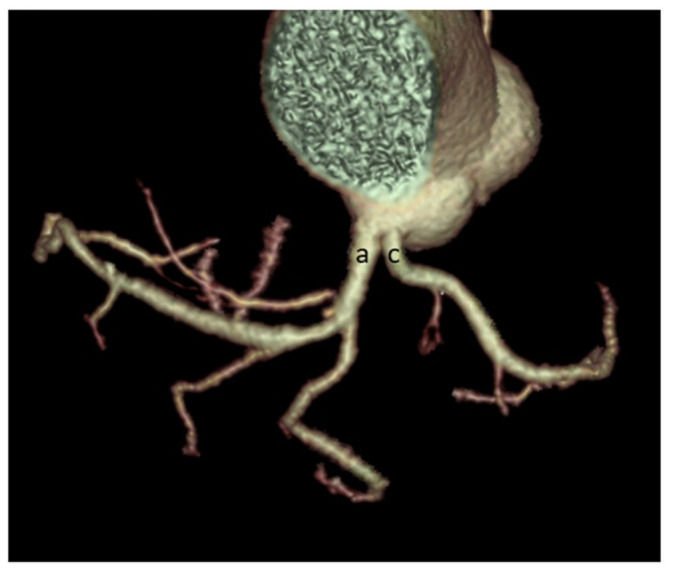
Separate origins of left anterior descending (a) and left circumflex (c) in computed tomography volume-rendering image.
